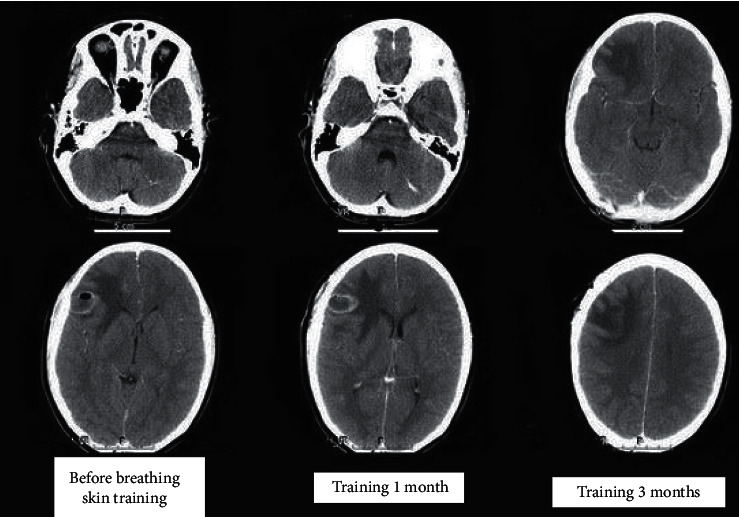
CT multislice spiral image with natural image processing (some images are from the Internet and have been authorized by the authors to be available).
